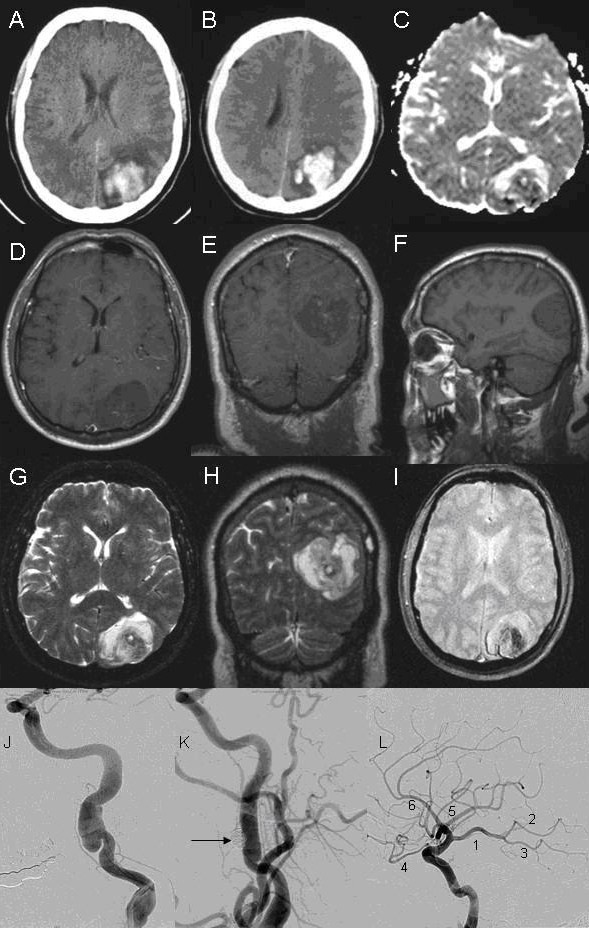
Neuroimaging of patient with occipital hemorrhage secondary to carotid dissection. A-B: Computerized tomography (CT) of the head showing left occipital hemorrhage. C: Diffusion weighted imaging (DWI). D, E, F: Axial, coronal, and sagital T1-weighted magnetic resonance imaging (MRI). G, H: Axial and coronal T2-weighted MRI. I: Gradient echo. J, K, L: Angiogram of left carotid artery. J: Left carotid angiogram showing pseudoaneurysm. K: Left carotid angiogram after deployment of coil (arrow) into pseudoaneurysm. L: Left internal carotid artery (ICA) angiogram with branches. 1: Fetal origin posterior cerebral artery (PCA) from ICA. 2–3: Anterior and posterior branches of PCA, respectively. 4: Ophthalmic artery. 5: Middle cerebral artery. 6: Anterior cerebral artery.
